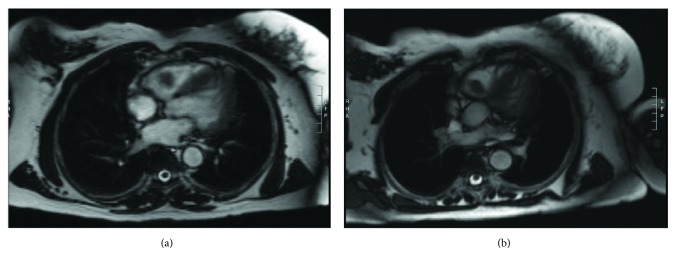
Cardiac magnetic resonance imaging showing an oblong soft tissue mass abutting the pulmonary valve.
